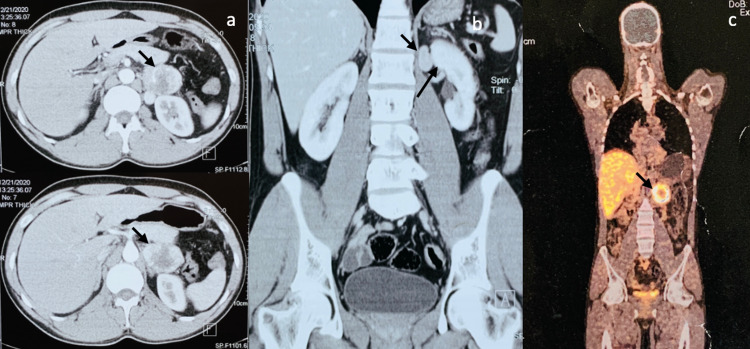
(a) and (b) Computerized tomography scan of the abdomen was ordered, which revealed a well-defined (35 x 46 x 55 mm) mass lesion in left peri-renal space with heterogenous intense arterial enhancement in close proximity to another similar homogenous lesion (20 x 17 x 32 mm), not seen separately from the left adrenal gland suggesting adrenal as well as extra-renal pheochromocytoma/paraganglioma. (c) Ga68-DOTANOC whole-body PET-CT similarly described the mass lesions expressing somatostatin receptors (SSTR). No other lesion was present elsewhere. DOTANOC - DOTA-(Nal3)-octreotide; PET-CT - positron emission tomography-computed tomography.
